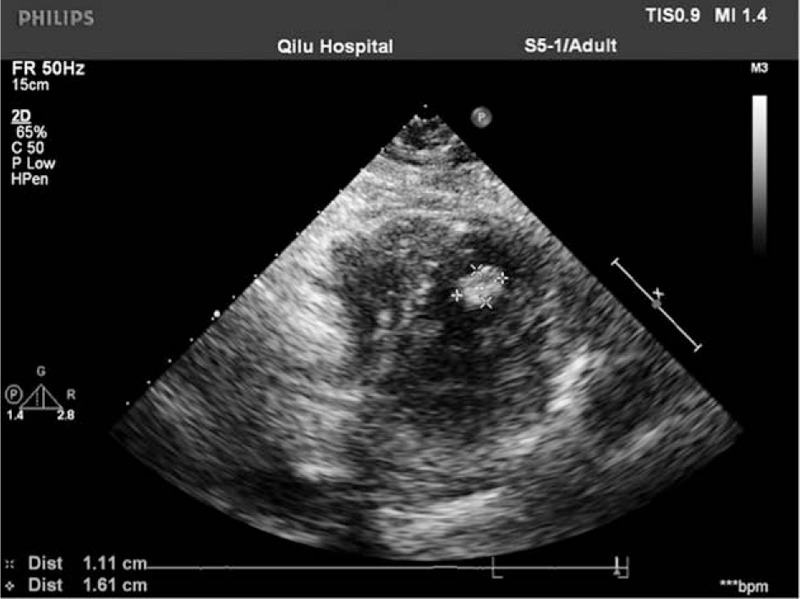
A preoperative transthoracic echocardiogram showed a hyperechoic mass in the left ventricle.
